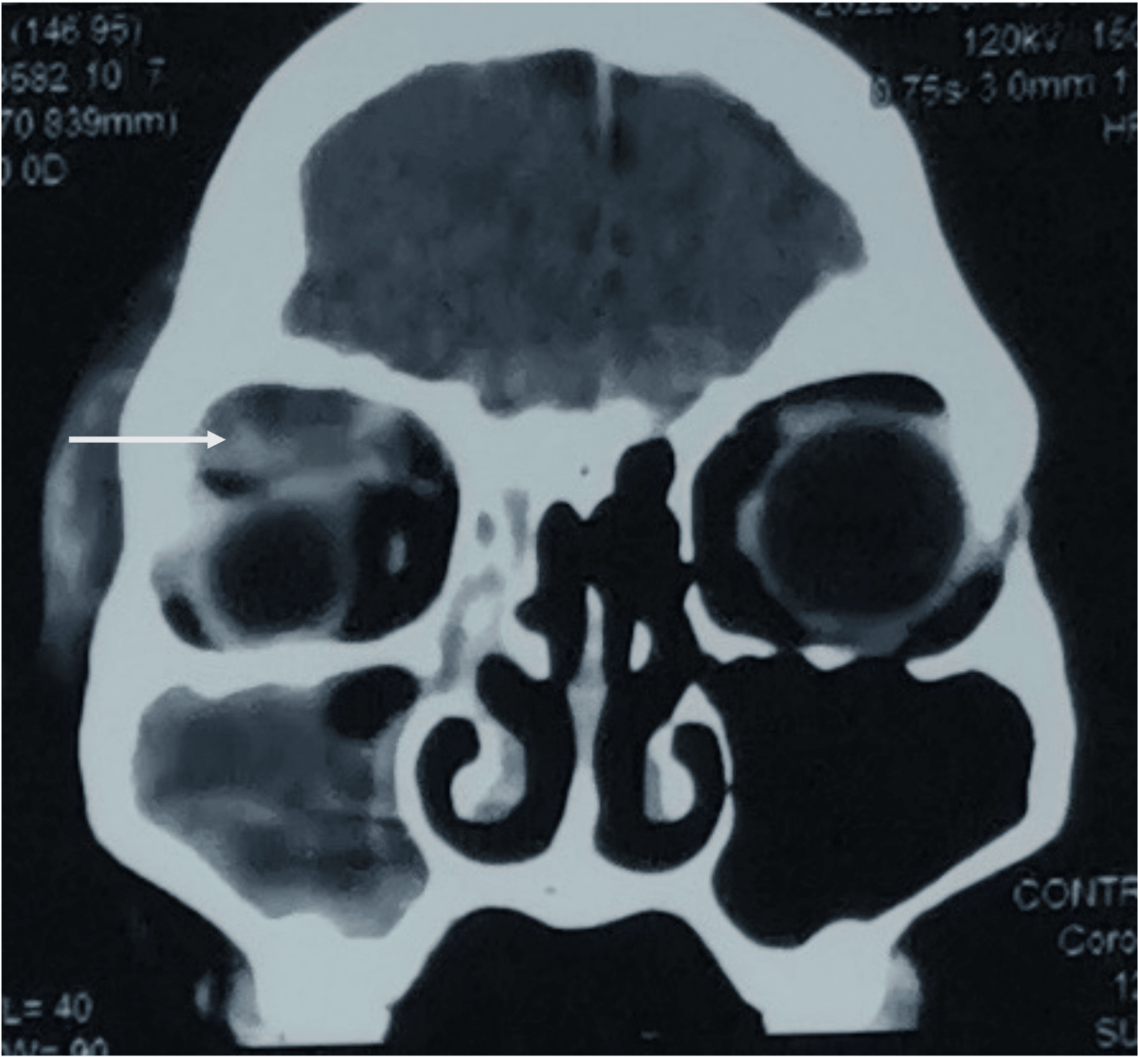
CT scan revealing a large abscess at the superior extraconal region (arrow) causing compression to the right eye globe.
